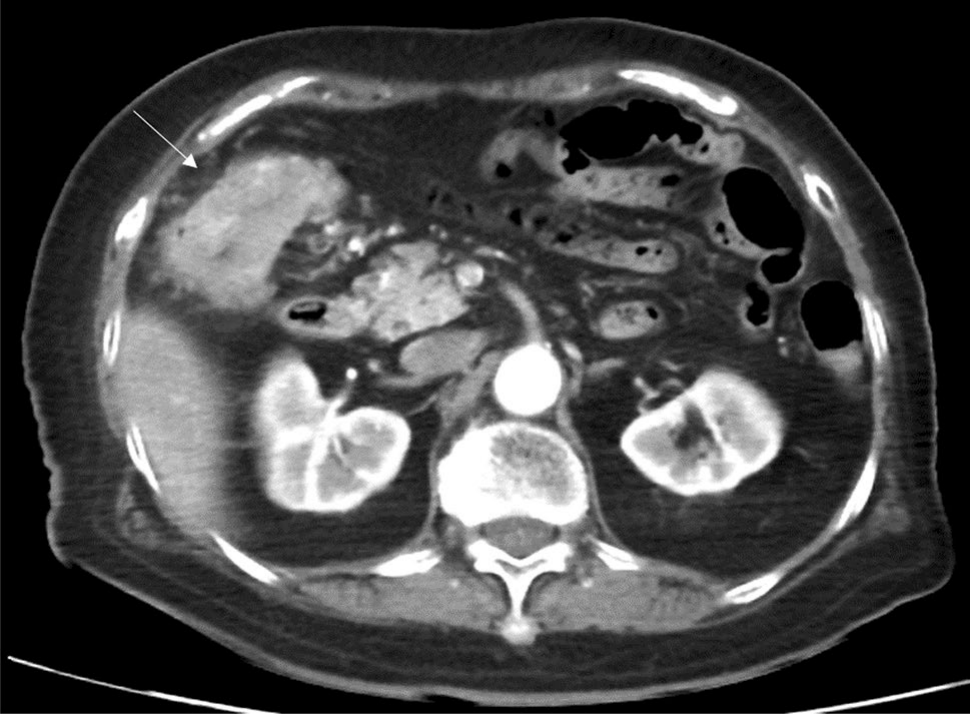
Enhanced computed tomography: wall thickening with enhancement at the hepatic flexure (arrow) without the findings suggesting lymph node metastasis, distant metastasis and intestinal bowel obstruction
